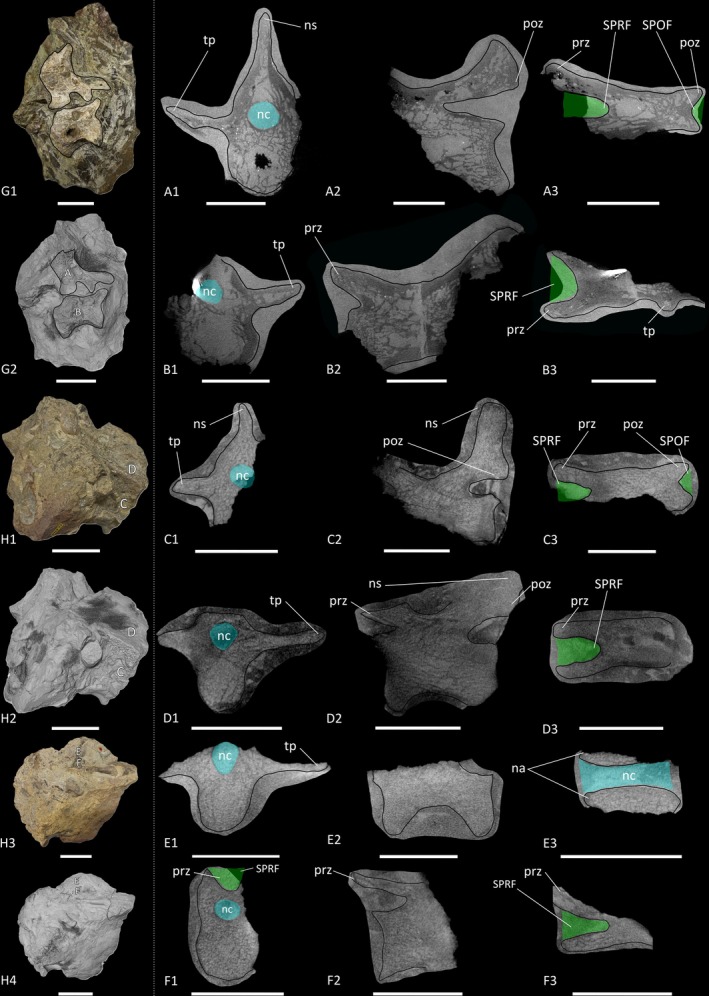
CT scan of *Thecodontosaurus antiquus* caudal vertebrae. (A1–A3) Element A, (B1–B3) element B, (C1–C3) element C, (D1–D3) element D, (E1–E3) element E, (F1–F3) element F; (A1–F1) CT scan transverse cross‐section, (A2–F2) CT scan parasagittal cross‐section, (A3–F3) CT scan horizontal cross‐section; (G1, G2) Cb 4164 block, (H1–H4) Cb 4714 block; (H1, H2) side 1, (H3, H4) side 2; (G1, H1, H3) photograph, (G2, H2, H4) surface scan. Na, neural arch; nc, neural canal; ns, neural spine; poz, postzygapophysis; prz, prezygapophysis; SPOF, spinopostzygapophyseal fossa; SPRF, spinoprezygapophyseal fossa; tp, transverse process. Key: Blue highlight, neural canal; green highlight, neural spine fossa. Scale bars = 20 mm (A–F), 50 mm (G, H).
